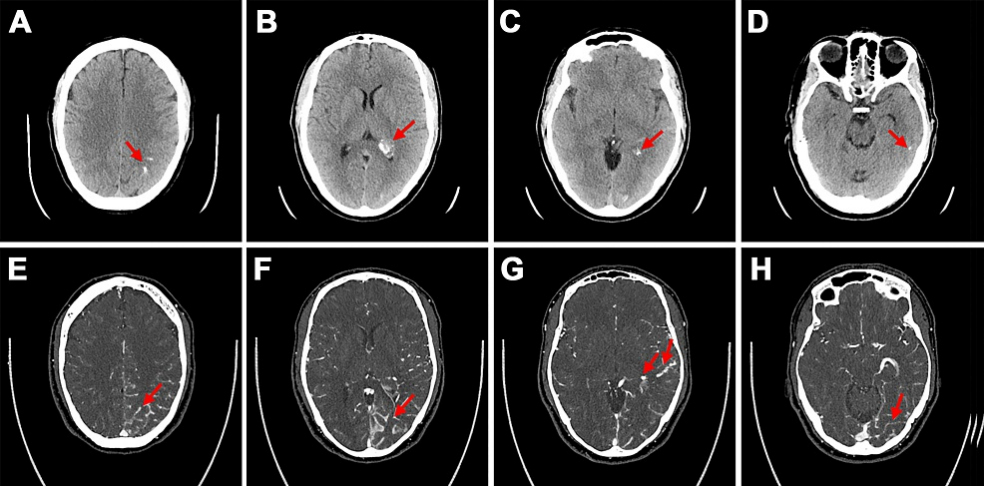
Diagnostic imaging Initial non-contrast head CTs (A-D) show curvilinear parenchymal calcification in the left parietal, occipital, and temporal lobes and asymmetric prominence and calcification of the left atrial choroid plexus (red arrows). Head CTA images (E-H) show prominent vascular structures insinuating along the left parietal, occipital, and temporal lobes consistent with a diagnosis of diffuse proliferative cerebral angiopathy (red arrows).
